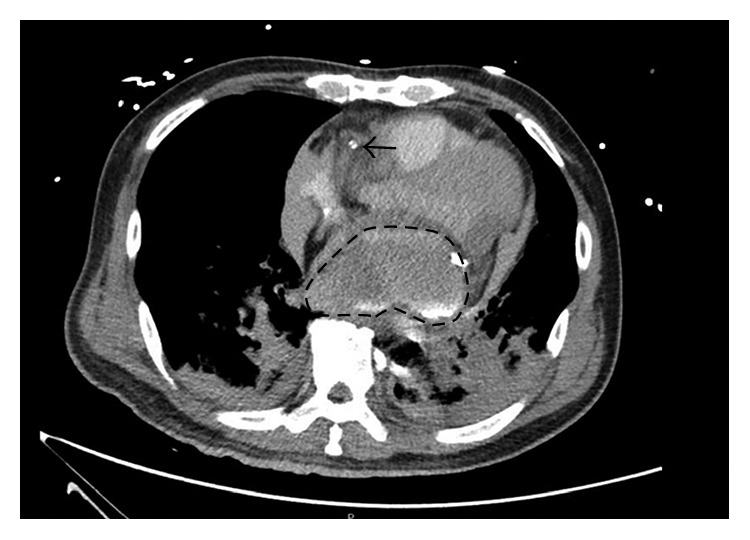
CT chest showing large hematoma arising from atrioventricular groove, with contrast from coronary angiogram, compressing on left atrium. The coronary sinus cannot be seen in the scan due to severe extrinsic compression. The arrow shows the ECMO cannula at the superior vena cava and right atrium junction.
